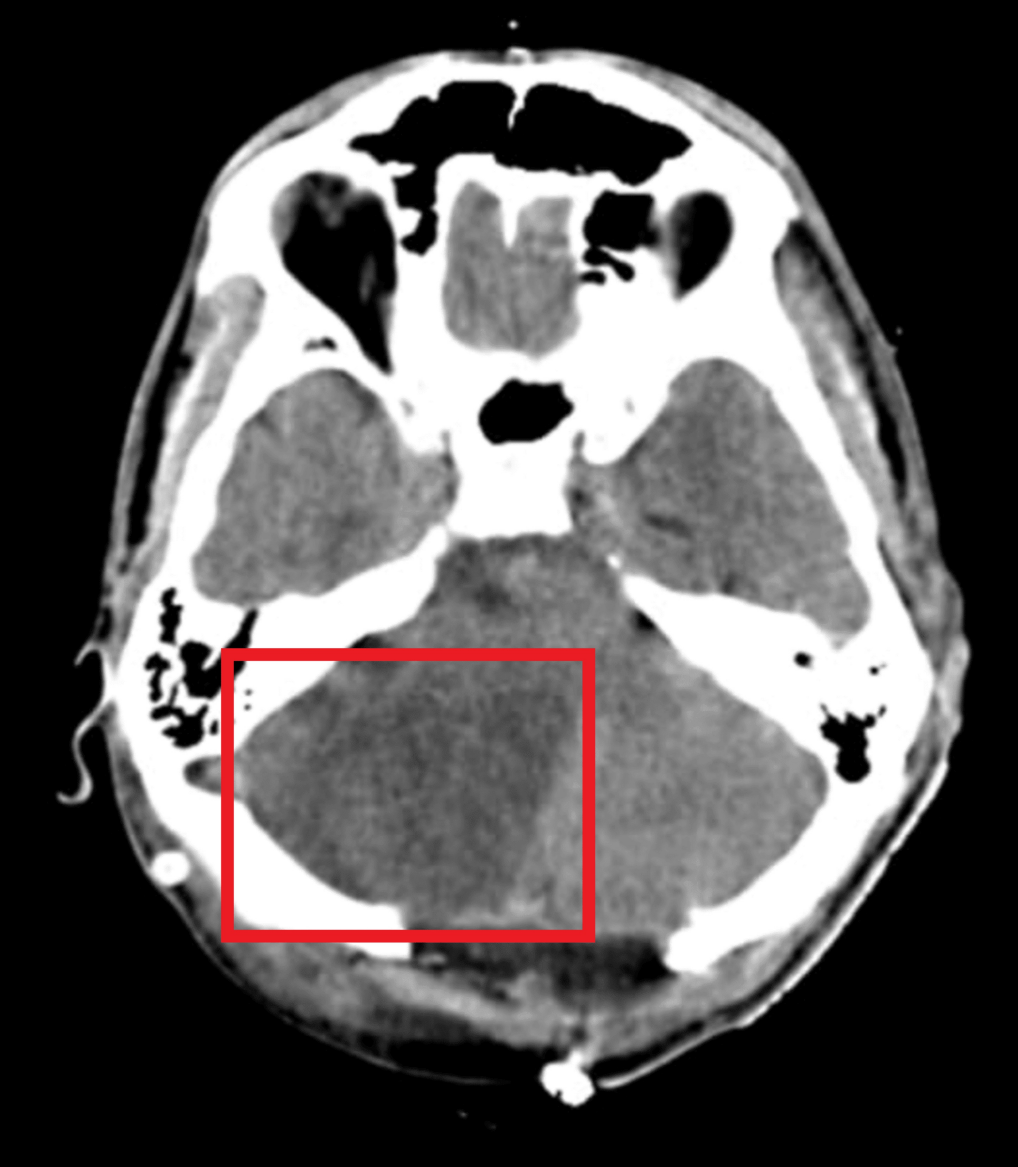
Magnetic resonance image suggestive of cerebellar infarct
